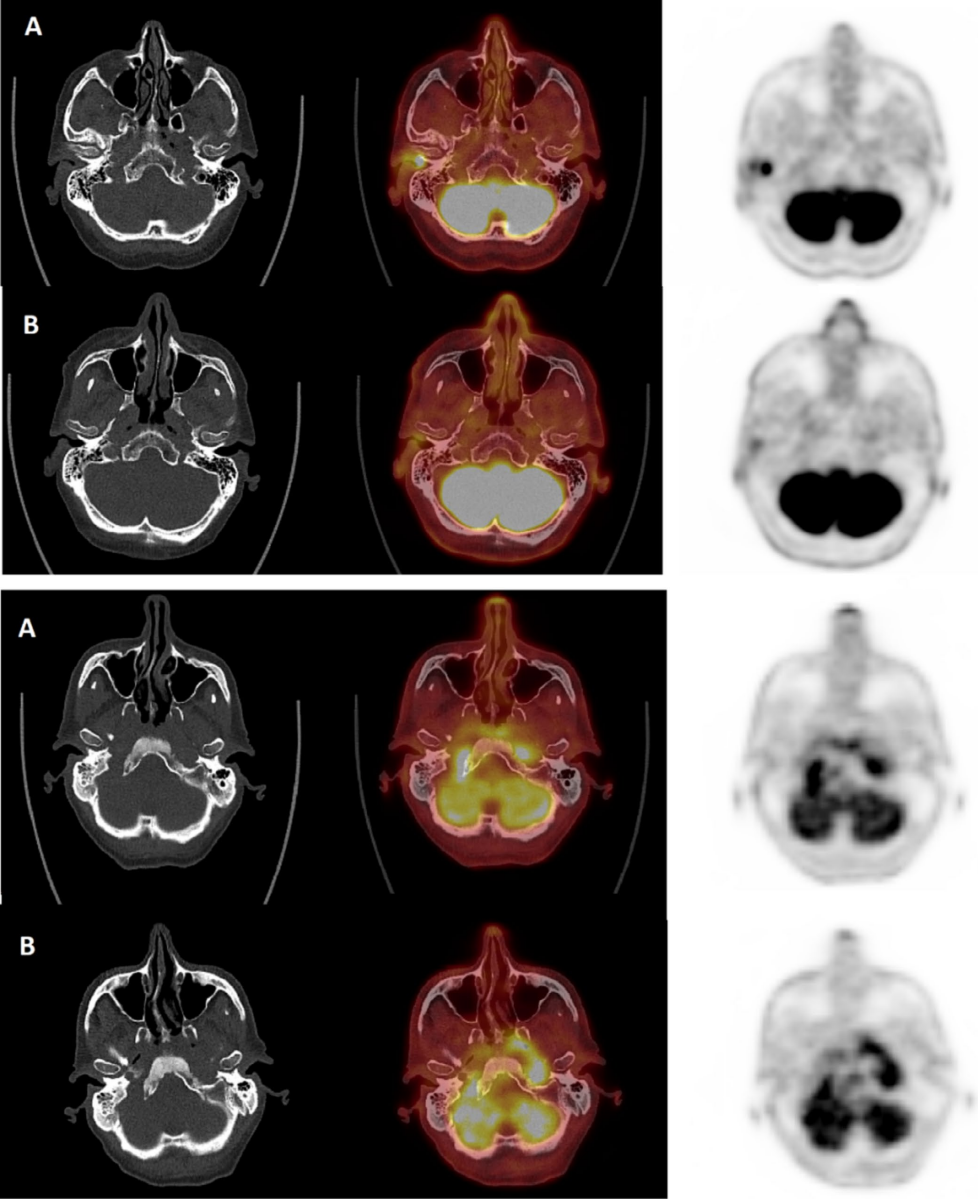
(a) Example of patient who have achieved a cure. A: initial PET scan; B: re‐evaluation PET scan; from left to right, in axial section: CT—PET‐CT fusion—PET alone. NOE with susceptible
